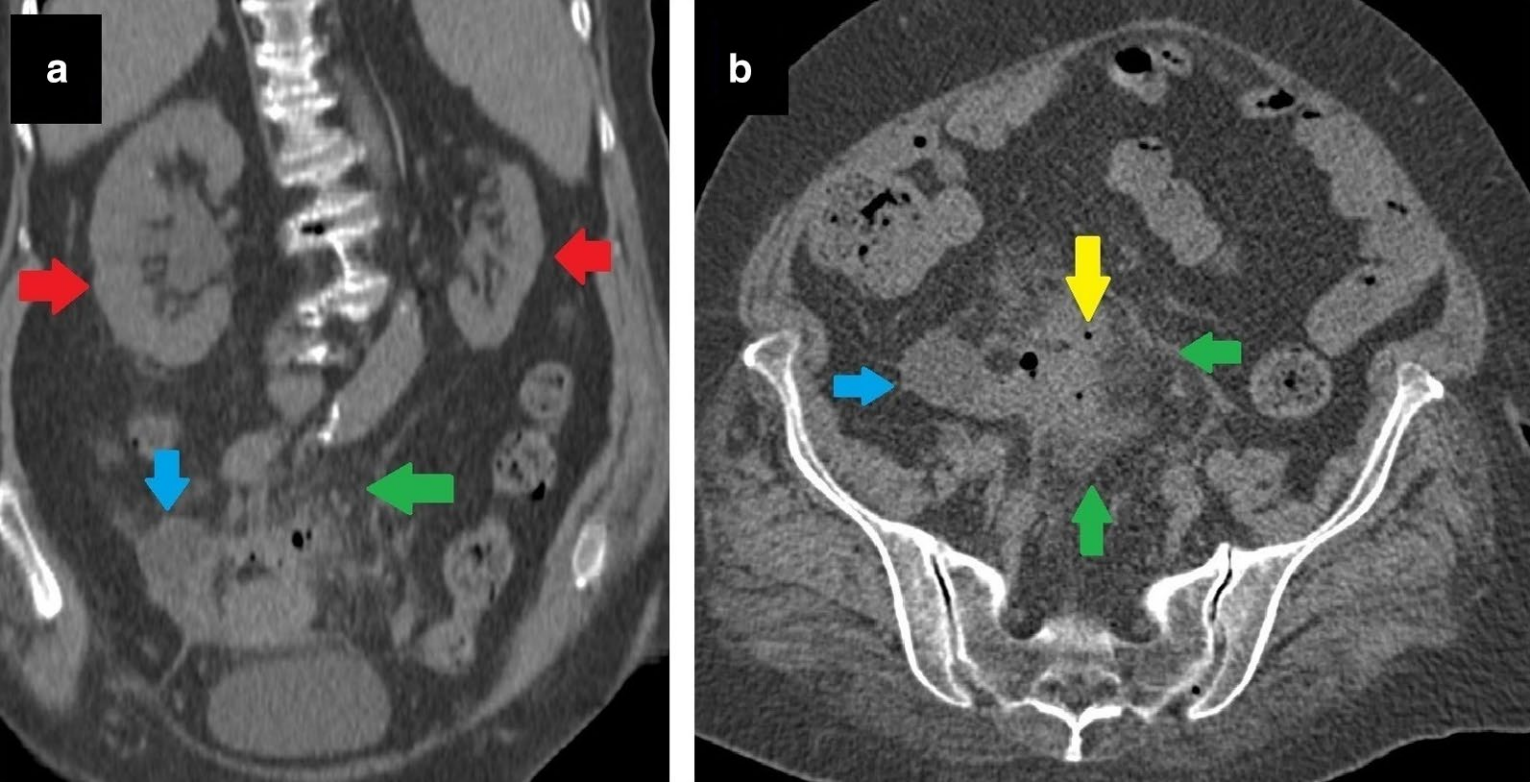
Framing bias causing an under-reading error. A 77-year-old female patient with a history of renal stone and recurrent urinary tract infections presented with lower abdominal pain. The patient’s symptoms did not regress under proper antibiotherapy. Non-enhanced CT was ordered for “evaluation of renal stones and urinary pathologies.” The CT was reported as “normal, except left atrophic kidney (red arrows, a).” However, a collection (blue arrows, a, b), free-air bubbles (yellow arrow, b) and mesenteric fat stranding (green arrows, a, b) adjacent to the sigmoid colon were overlooked due to the framing of clinical information. Although non-enhanced CT was technically inadequate to evaluate bowel pathologies, inflammatory changes were clearly visible on CT, and at least a suspicion should have been expressed. During follow-up, lower abdominal MRI revealed the final diagnosis as “perforated sigmoid colon malignancy” (not shown)
